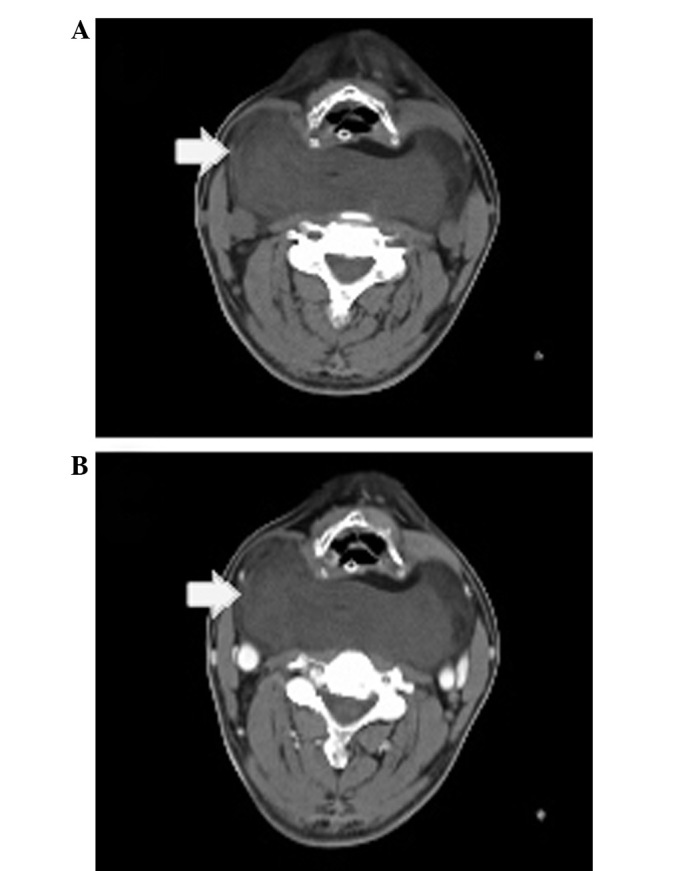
Computed tomography (CT) scans. (A) Retropharyngeal space occupied by an extremely large mass (arrow) extending to the sides of the neck. (B) The mass (arrow) was not enhanced following contrast agent administration.
